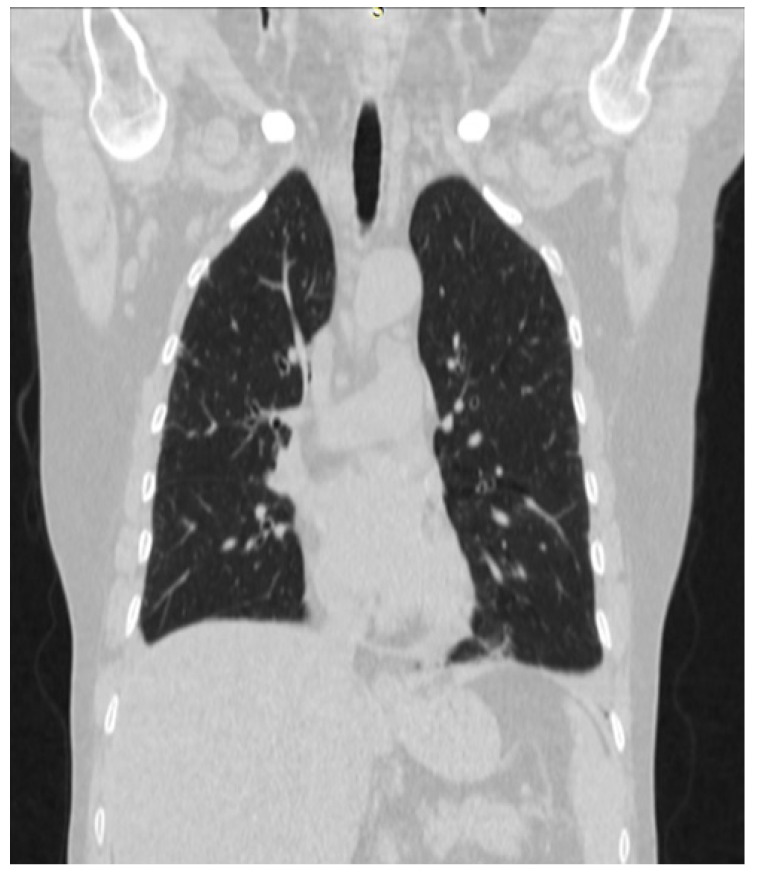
HRCT showing NSIP (frontal section).
